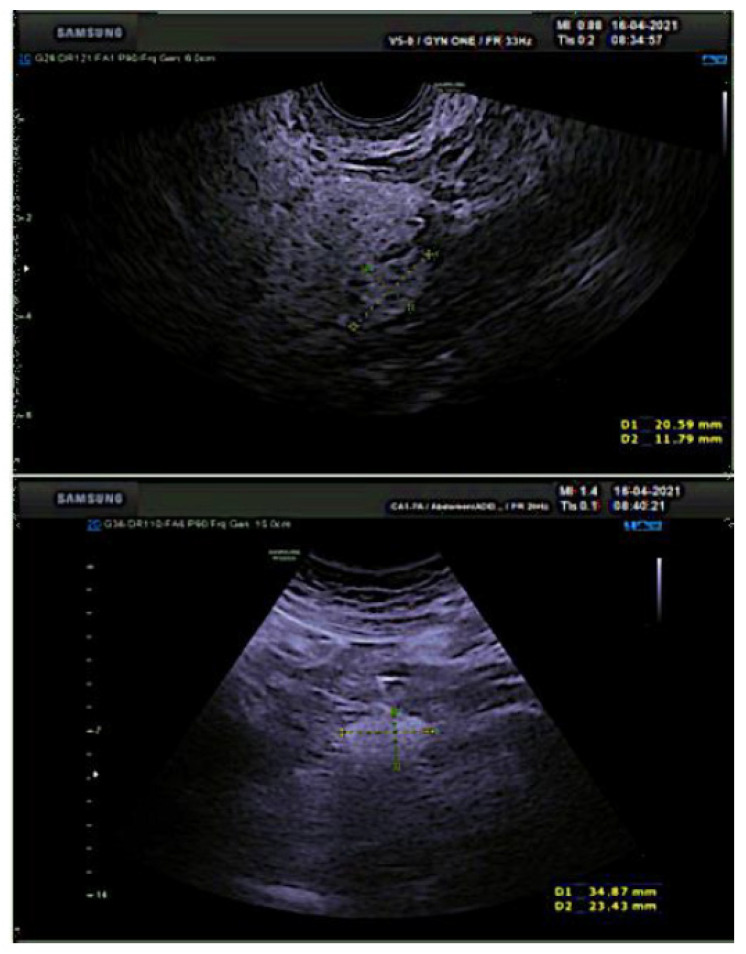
Ultrasound preoperative image showing isolated lymph node recurrence (ILNR) 20 × 34 × 23 mm in size, attached to the external iliac vessels.
